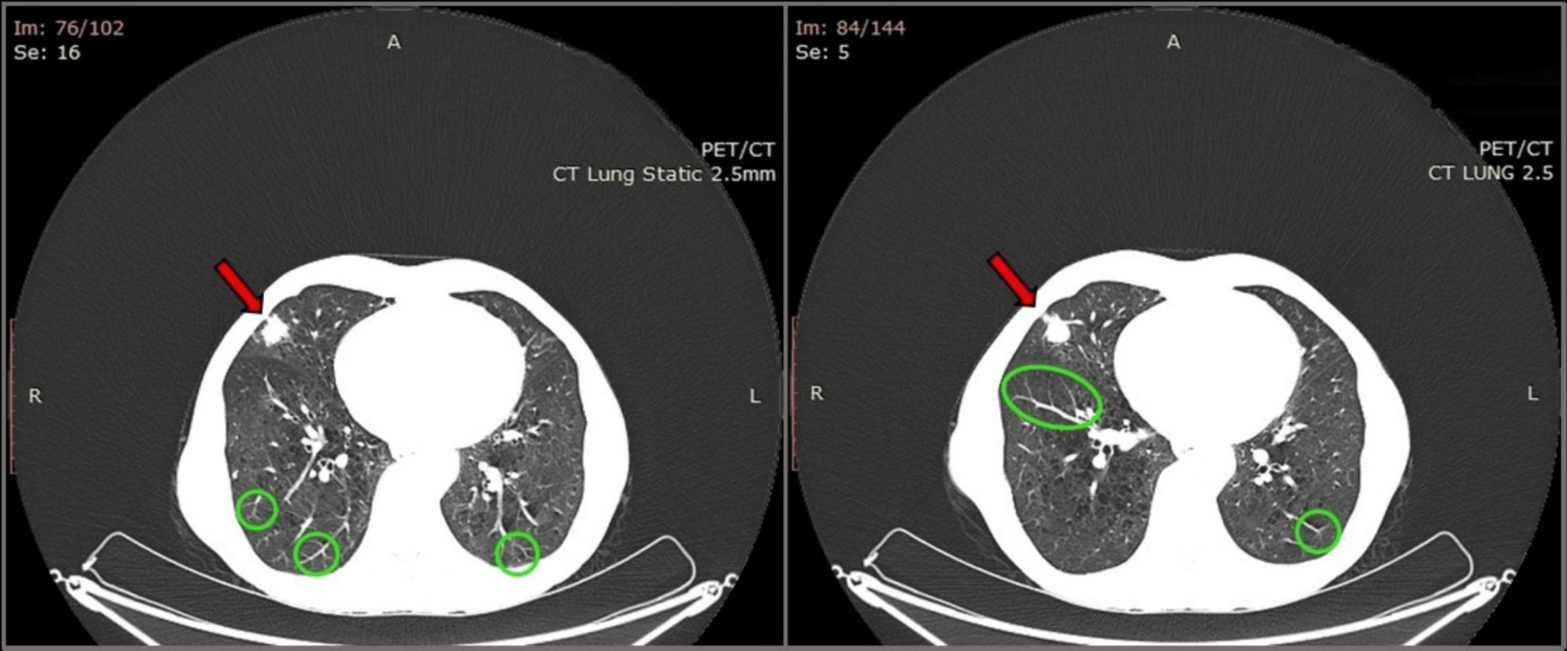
CT Scan of the Lungs Revealing a Middle Lobe Nodule With Multiple Micronodules Chest CT scan lung window images reveal a noncalcified nodular lesion with irregular margins, measuring 18 mm in diameter, located in the lateral segment of the middle lobe, highly suspicious for lung cancer (red arrows). Furthermore, multiple bilateral micronodules, along with tree-in-bud opacities, are observed (green circles) PET/CT: positron emission tomography/computed tomography
